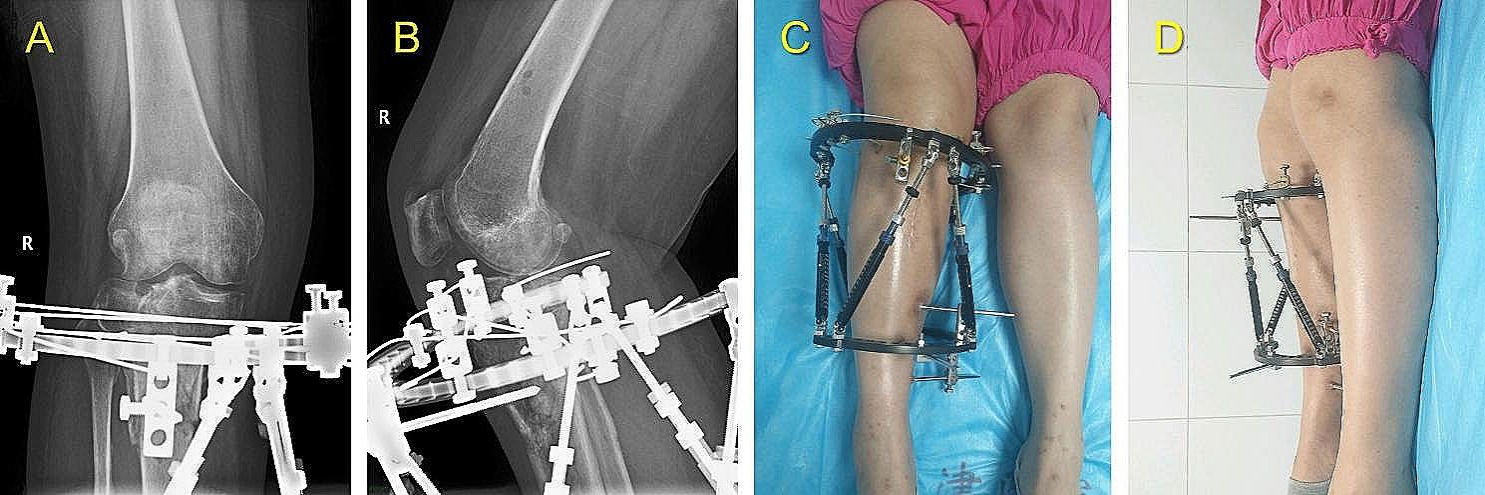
The last follow-up images of the same patient shown in the Fig. 5 before removing the TSF. (A, B) AP and lateral views of X-rays 24 weeks after surgery. (C, D) Clinical AP and lateral views 24 weeks after surgery
